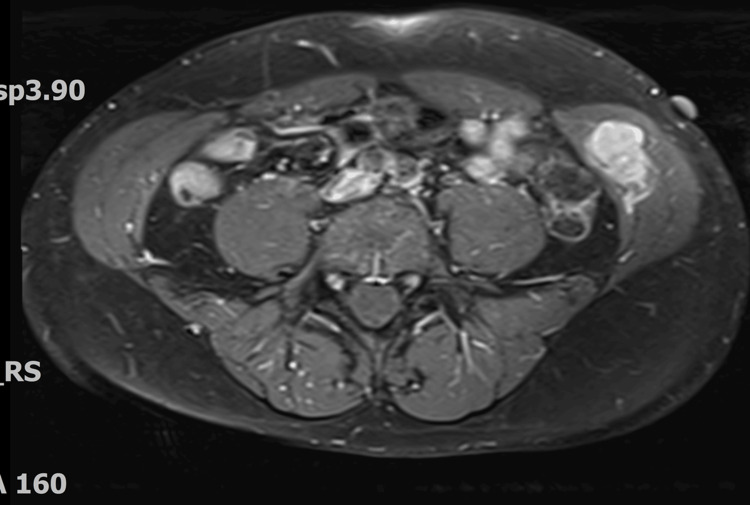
MRI post-contrast T1 with FAT-SAT (fat-saturation) Space-occupying mass with enhancement measuring 3.0 cm in the greatest dimension occupying the left Internal oblique muscle.
